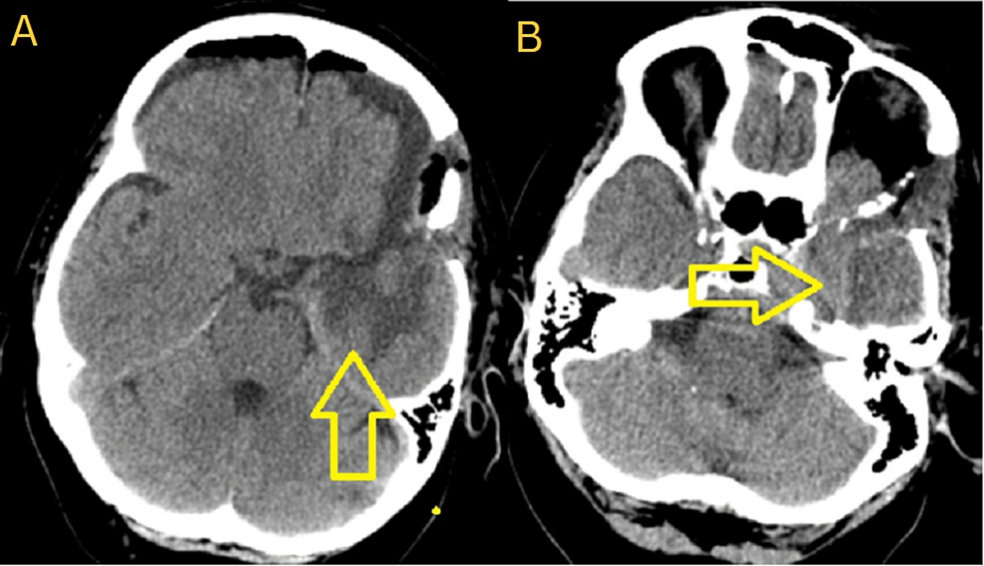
Postoperative CT scan. А - Аxial plane. Subtotal resection. No evidence of ischemic or hemorrhagic events. B - Axial plane. Subtotal resection. No evidence of ischemic or hemorrhagic events.
